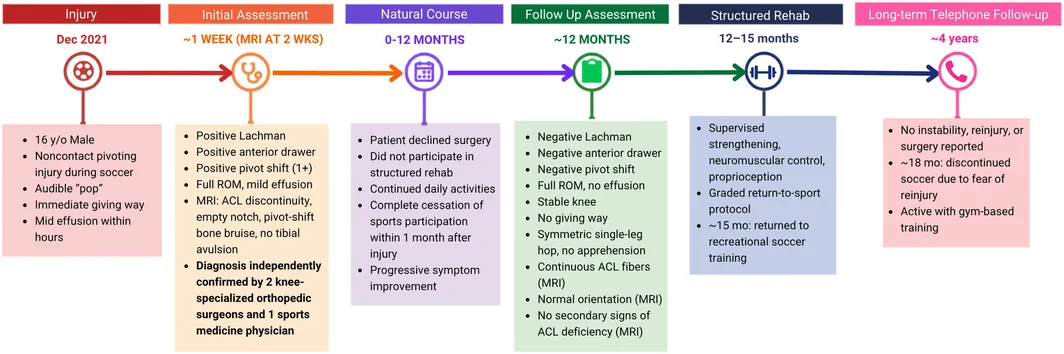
Chronological sequence of injury, assessment, management, and outcome. A 16‐year‐old soccer player sustained a noncontact pivoting injury with clinical and MRI evidence of functional complete ACL rupture. After declining reconstruction, he improved without surgery. At ~12 months, clinical examination was stable and MRI showed restored ACL continuity. Structured rehabilitation and graded return to sport followed, with return to soccer training at ~15 months. Telephone follow‐up at ~4 years revealed no reported instability, reinjury, or surgery; he had discontinued soccer because of fear of reinjury and remained active with gym‐based training.
